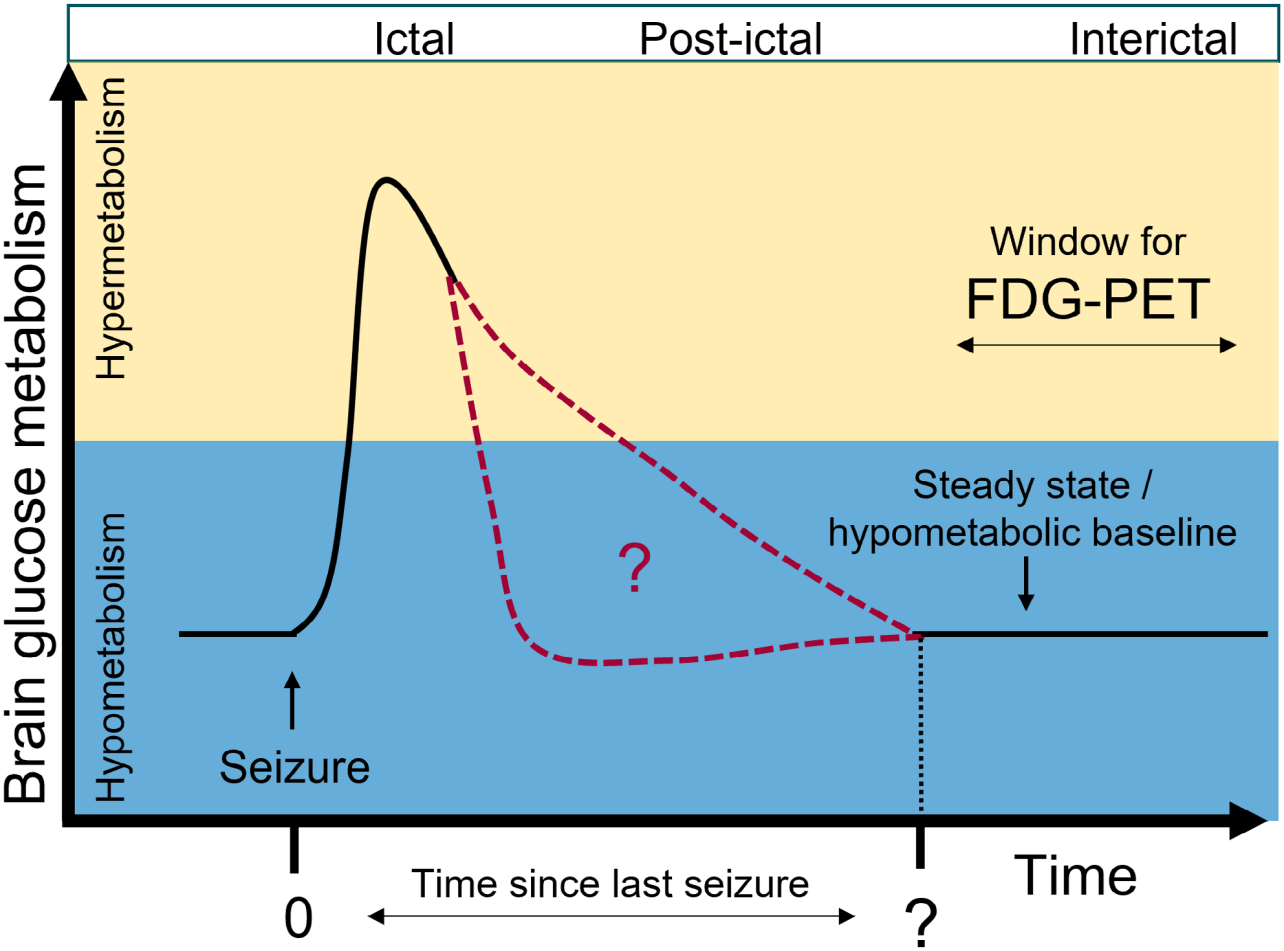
Graphic conceptualization of dynamic brain glucose metabolism following an epileptic seizure (time = 0) in epileptogenic foci. The interictal hypometabolic (blue area) baseline is disturbed by a seizure resulting in temporary hypermetabolism (yellow area). The progression of the brain glucose metabolism is unknown (red dotted line) as is the time since last seizure to the hypometabolic steady state. Abbreviation: FDG‐PET, fluoro‐2‐deoxyglucose positron emission tomography
